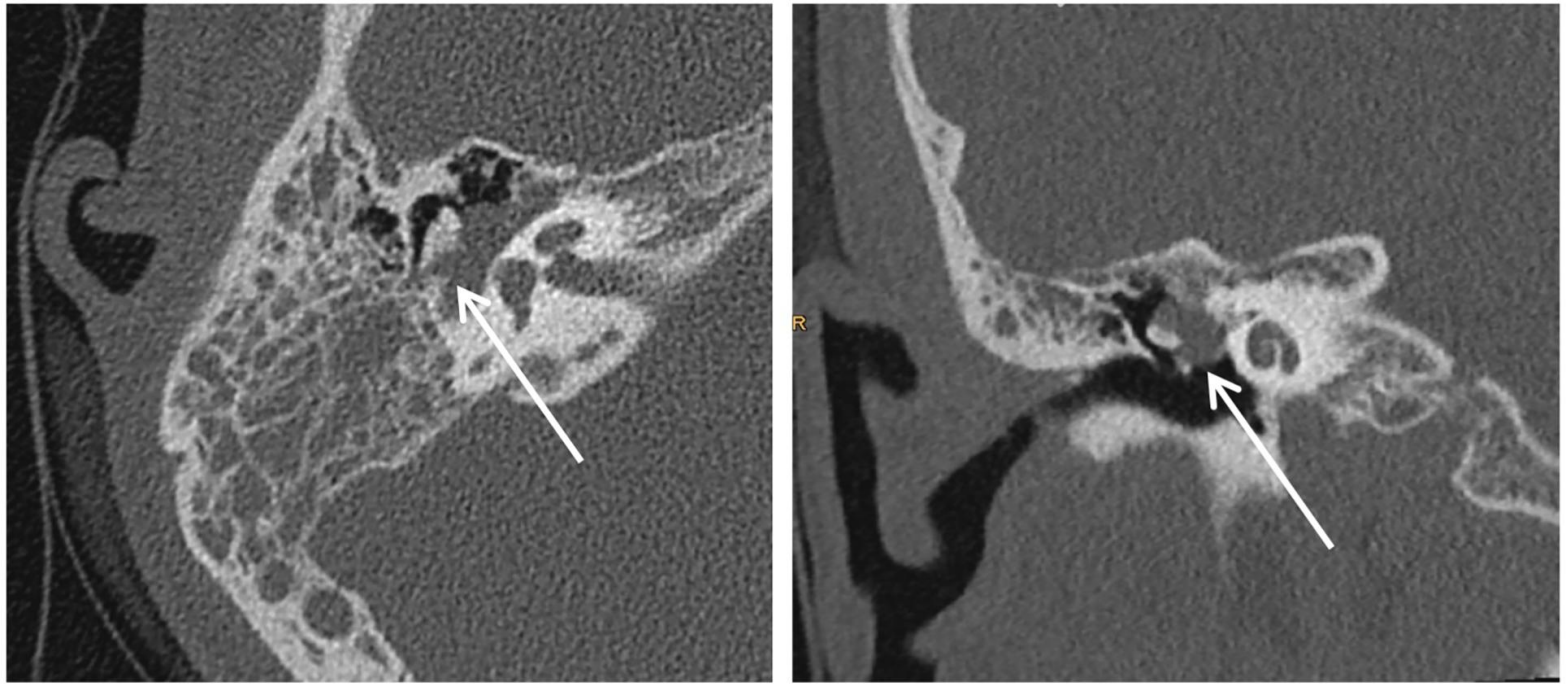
CT scan of the right temporal bone (right axial and left coronal): soft tissue opacity of the right mesotympanum and hypotympanum (arrows). No signs of erosion of the ossicles. Complete opacification of the air cells of the right mastoid.
